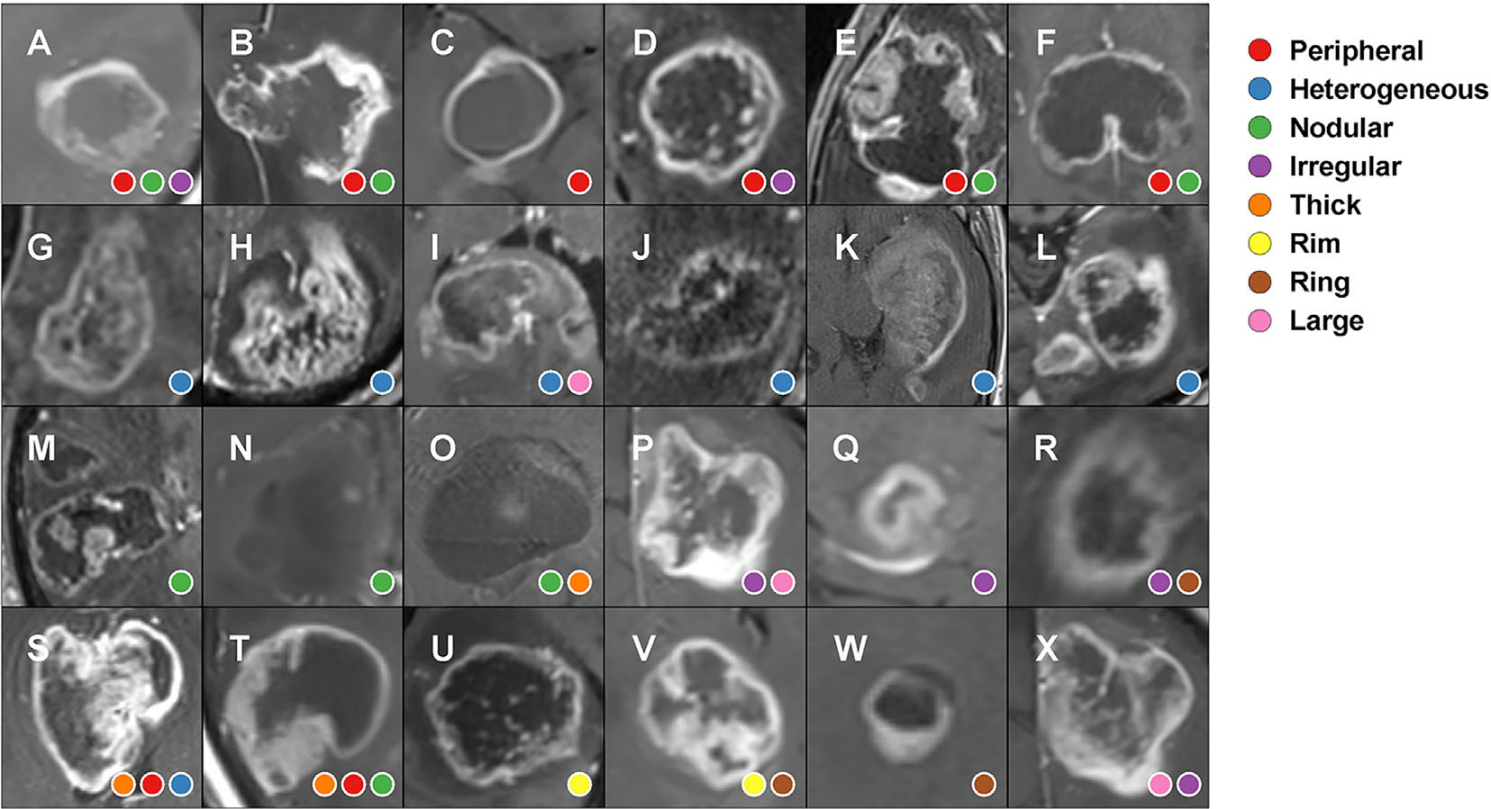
Glioma enhancement patterns and how radiologists described them in pre-operative MRI reports. (A–F) peripheral enhancement; (G–L) heterogeneous enhancement; (M–O) nodular enhancement; (P–R) irregular enhancement; (S, T) thick enhancement; (U, V) rim enhancement; (W) ring enhancement; (X) large enhancement.
